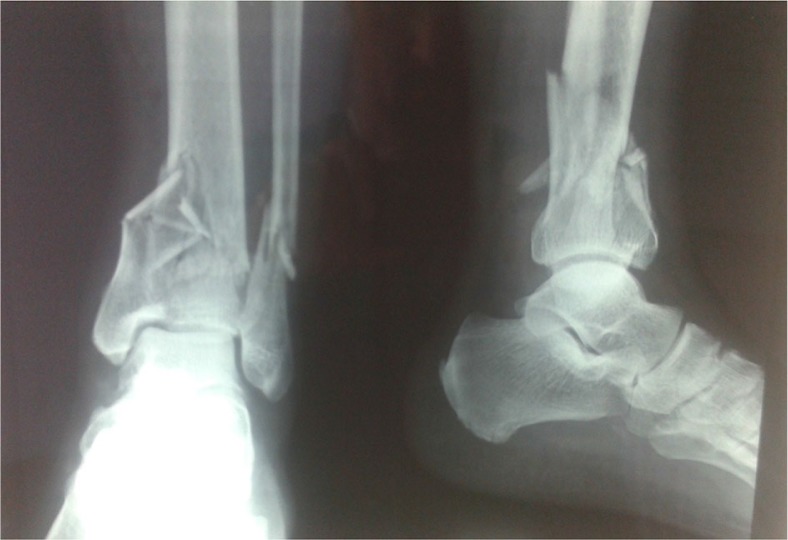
Initial lower extremity radiographs showing the distal tibial and fibular fractures.
